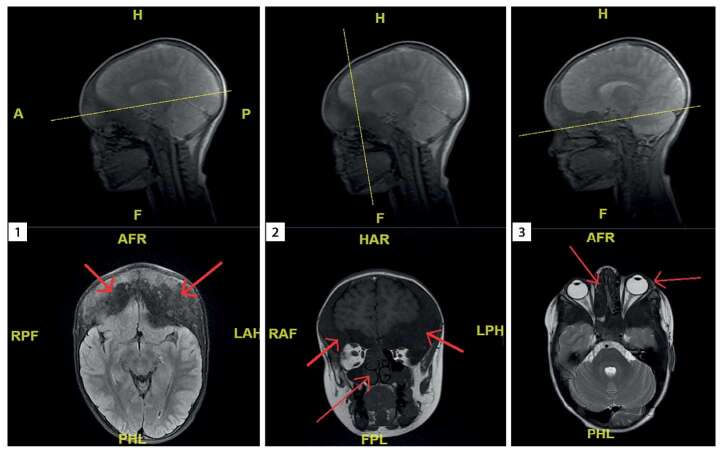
Рисунок 2. МРТ головного мозга в трех срезах.Срез 1 — утолщение лобных костей, облитерация лобных пазух. Срез 2 — утолщение лобных костей, облитерация правой верхнечелюстной пазухи справа, искривление носовых перегородок, сужение носовых ходов. Срез 3 — проптоз глазных яблок, облитерация верхнечелюстной пазухи справа, искривление носовой перегородки.Figure 2. MRI of the brain in three sections.
